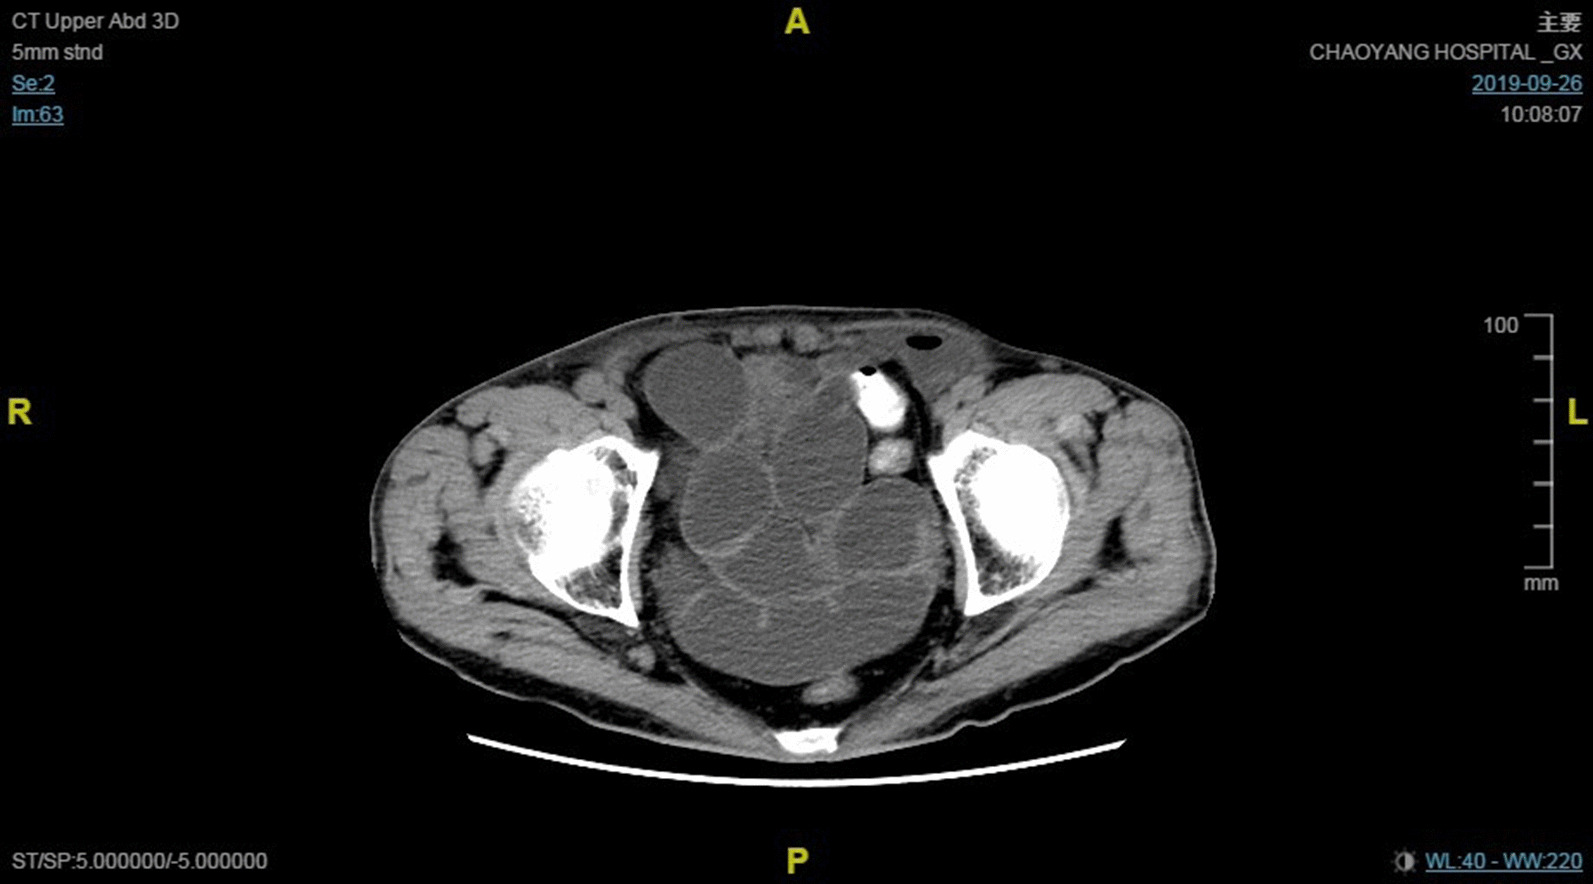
CT scans showed multiple distended bowel loops, exudates in both groin areas, and air-fluid levels in the left preperitoneal space
